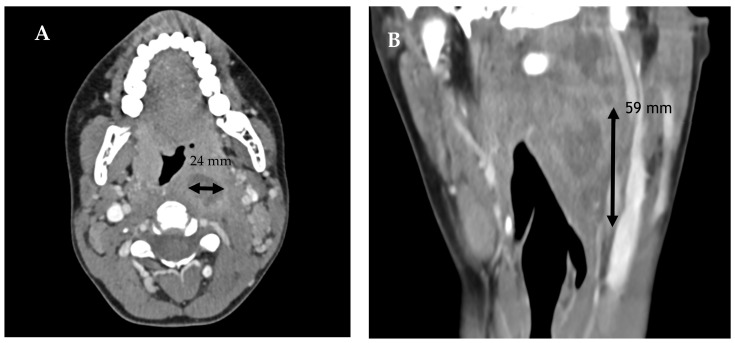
Case 4. Axial (A) and coronal (B) contrast-enhanced CT scans show a left retropharyngeal abscess, with multiple coalescent liquefactive areas, measuring 16 × 24 mm on the axial plane and 59 mm craniocaudally, protruding into the oropharyngeal lumen.
